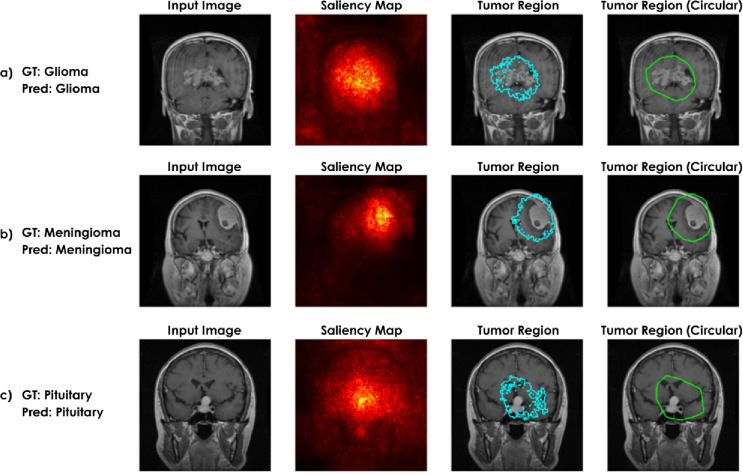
Examples of correctly localized tumor regions validated by a radiologist. Each row represents a different tumor type (top to bottom: glioma, meningioma, pituitary). The columns are the input MRI image, the saliency map generated by Hybrid Saliency, the raw tumor region correctly discovered and the smoothened tumor region for better visualisation.
